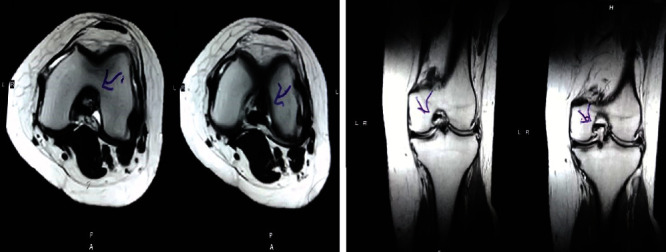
Posttreatment MRI showing improvement in the tear in the posterior horn of the medial and lateral menisci. The patient experienced symptomatic improvement as well as enhanced ability to perform her activities of daily living.
